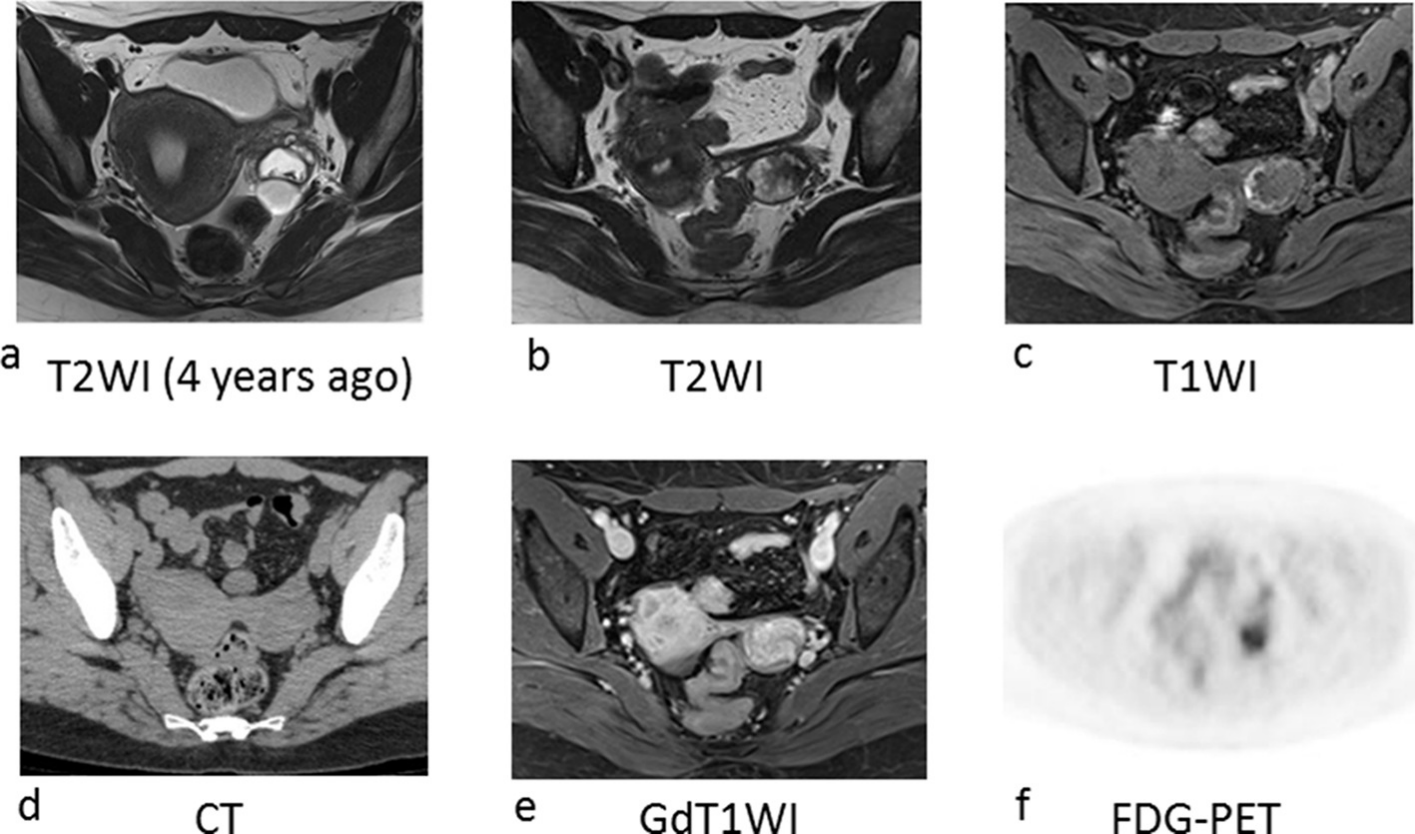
Imaging of Case 4. (a) MRI 4 years before the operation. The content of the cyst in the left ovary showed high intensity on T2WI with *T*_2_ dark spot sign and intermediate-to-low intensity on T1WI (not shown) indicating endometriotic cyst. (b) MRI 1 month before the operation. On axial T2WI, small tumour is observed at the left side of the uterus. There was papillary projections with low signal intensity were observed within the cyst. Haemorrhage is observed at the peripheral of the tumour on T1WI (c) and CT (d). Papillary projections within the cyst were well enhanced on gadolinium enhanced T1WI (e) and shows strong uptake at FDG-PET (f). FDG-PET, fluorodeoxyglucose positron emission tomography; T1WI, *T*_1_ weighted image; T2WI, *T*_2_ weighted image.
